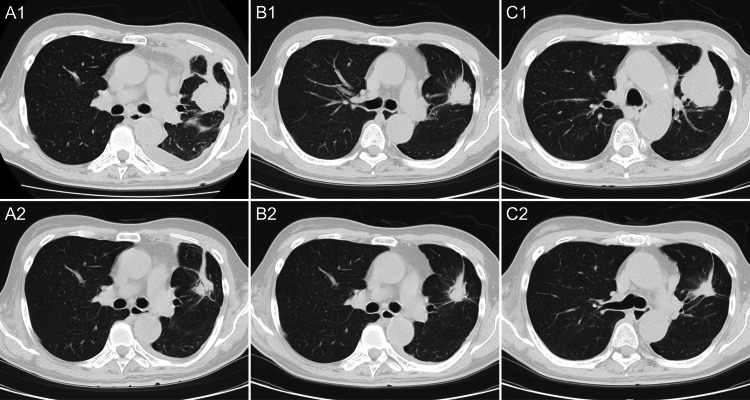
Serial chest CT images showing tumor changes during treatment. Responses were assessed per RECIST version 1.1. (A1) Before initiation of osimertinib. (A2) Partial response after initiation of osimertinib. (B1) Disease progression after osimertinib and before initiation of ICI-based therapy. (B2) Stable disease after initiation of ICI-based therapy. (C1) Disease progression after ICI-based therapy and before initiation of afatinib. (C2) Partial response after initiation of afatinib. CT, computed tomography; ICI, immune checkpoint inhibitor; RECIST, Response Evaluation Criteria in Solid Tumors.
